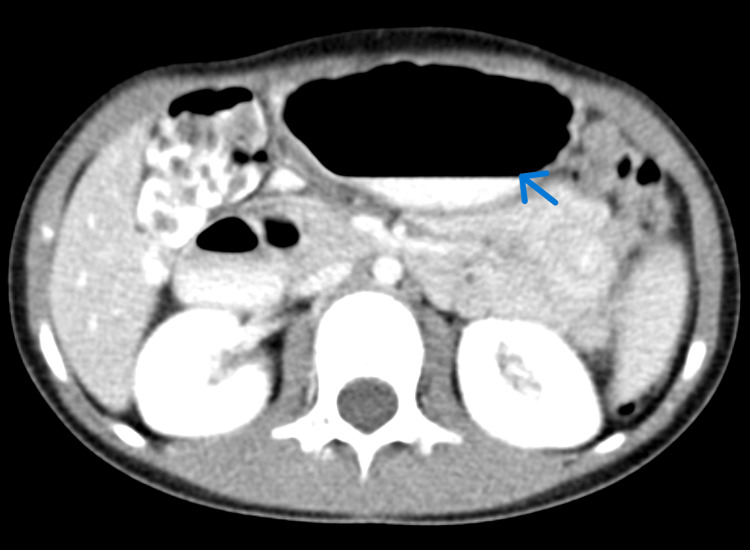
Axial CT showing dilated proximal duodenum with an air-fluid level
